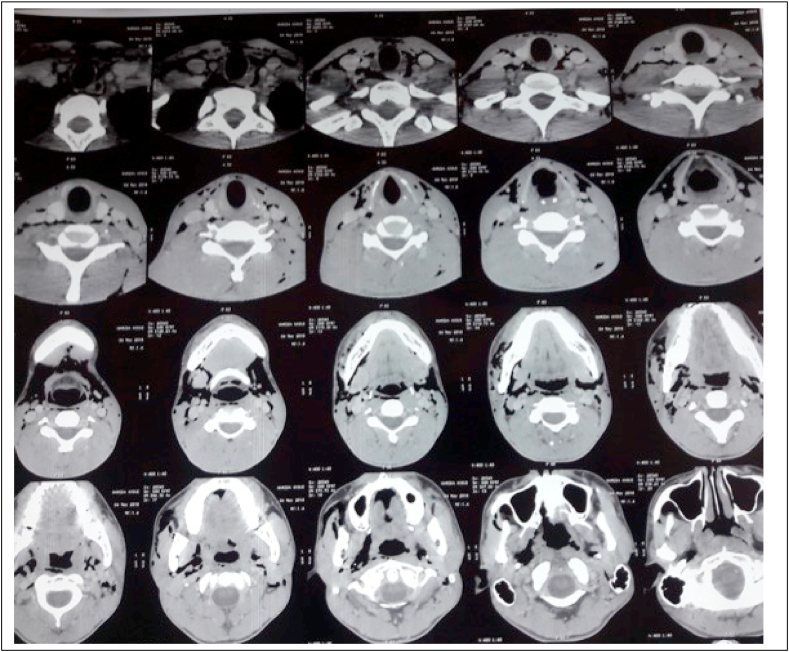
Cervicofacial CT showing emphysema of the deep facial and soft tissue spaces.
